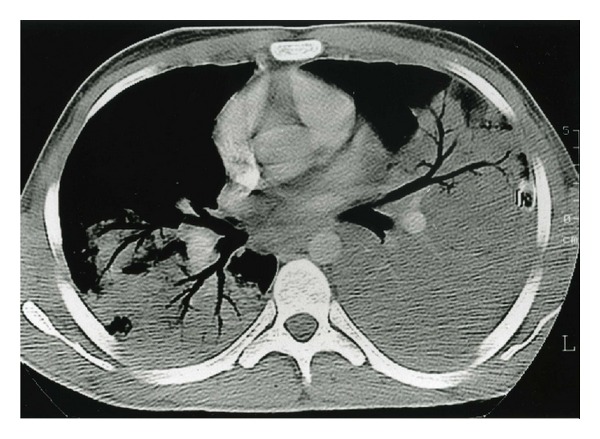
Thoracic CT scan on the 7th day after hospitalization showing a bilateral pneumonia with pleural effusion.
